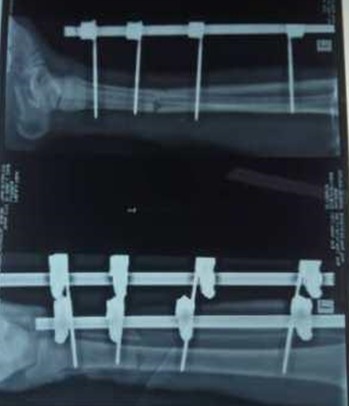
X-ray of the limb after debridement and application of external fixator
